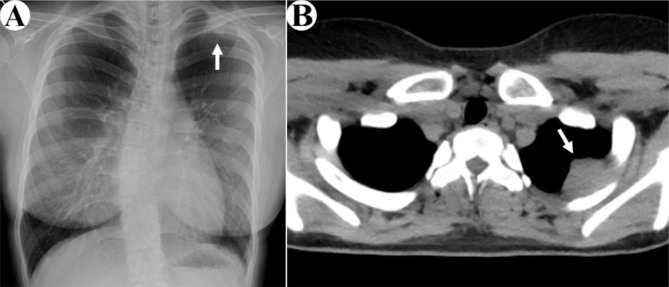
Preoperative radiological imaging. a: Chest radiography shows an abnormal shadow in the left upper lung field, representing the extrapleural sign (white arrow). b: Chest computed tomography shows a 29-mm nodule in the left second intercostal space (white arrow)
